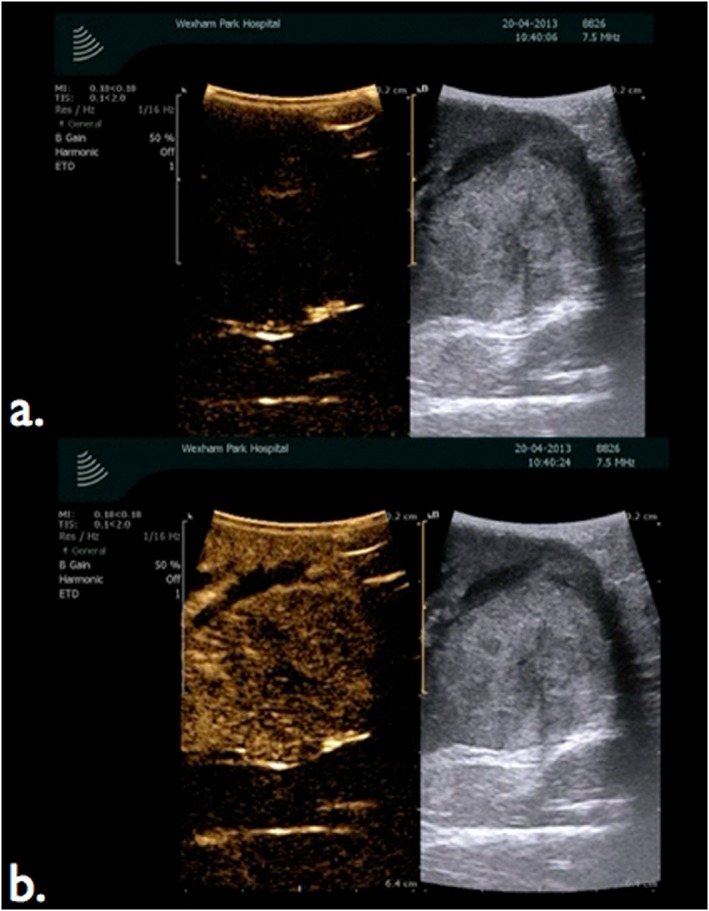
In situ* mapping of renal blood flow using B mode ultrasound image of renal tumour (right) with contrast enhanced ultrasound mode (left). (a) Prior to injection of SonoVue or with selective occlusion of a branch of the renal artery supplying the region of the tumour or (b) increase in echogenicity of tumour and renal parenchyma 20 s after intravenous injection of SonoVue demonstrating blood flow when the branch of the renal artery supplying the region of the tumour is not clamped
